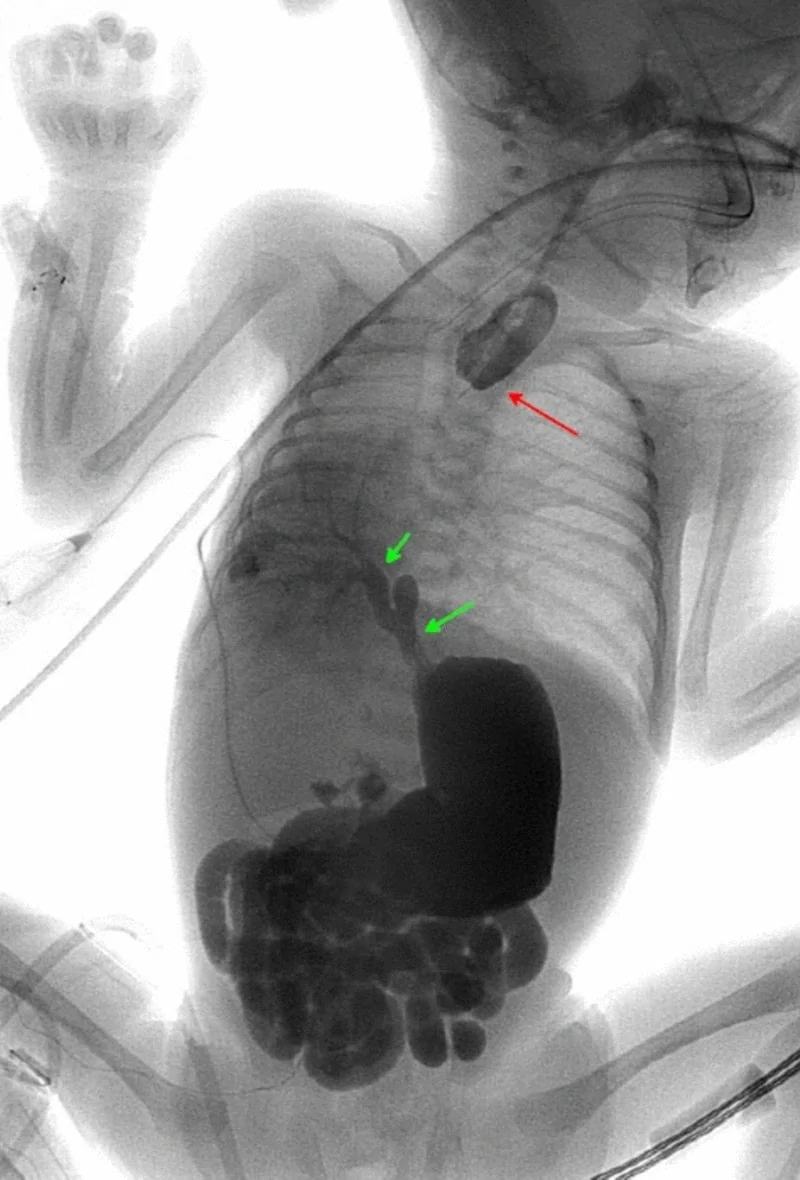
Preoperative Gastrografin study (aged 29 days). An anterior–posterior radiograph demonstrating contrast administration through a gastrostomy tube and a nasogastric tube. The nasogastric tube terminates in a dilated, blind-ended upper esophageal pouch in the upper thoracic region, consistent with esophageal atresia (EA). Following Gastrografin administration, an abnormal tract is opacified. This tract appears to communicate with an atretic/hypoplastic right lung, where the contrast outlines a branching pattern resembling the bronchial tree. These findings are highly suggestive of congenital EA with a bronchoesophageal fistula, likely associated with pulmonary aplasia/hypoplasia of the right lung. The radiological features support a complex congenital foregut malformation and demonstrate the utility of contrast studies for delineating aberrant fistulous communications in the neonatal period
